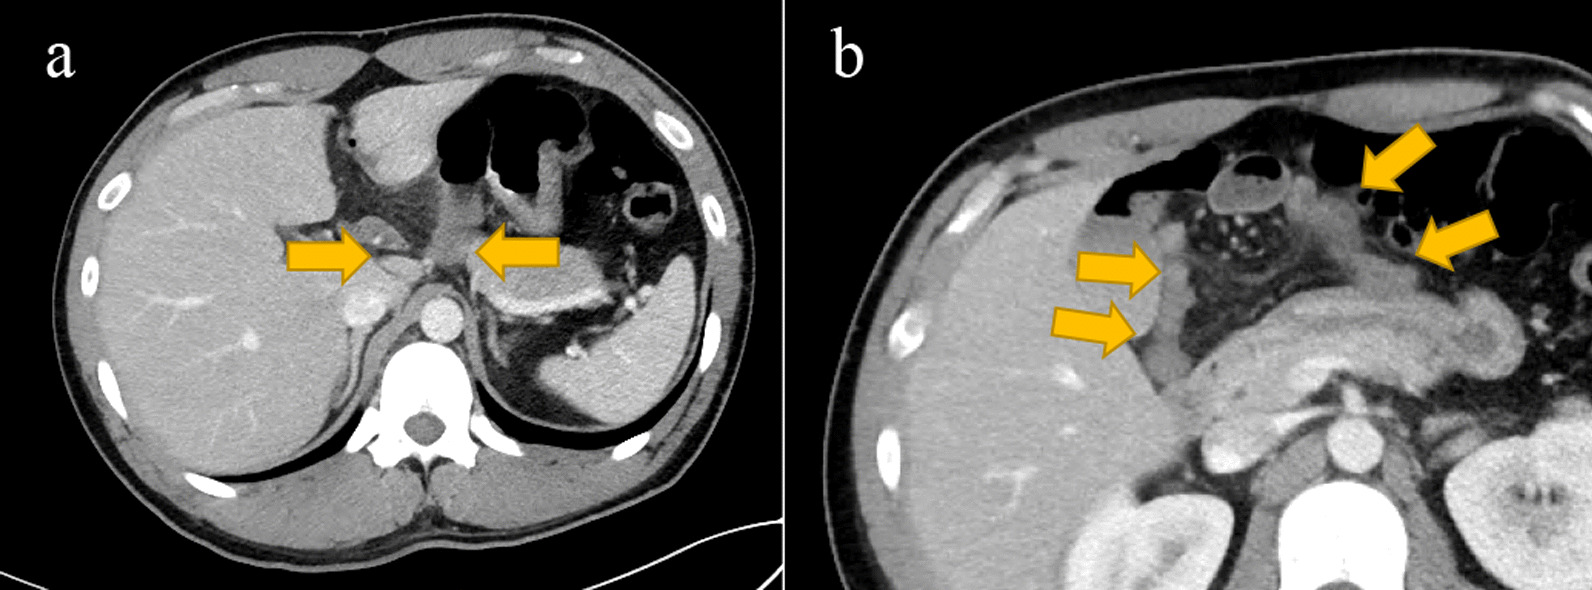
Contrast-enhanced computed tomography (CT) imaging. a The formation of a closed loop with a hernia portal near the hilar region. b Poor contrast of the prolapsed small intestine
